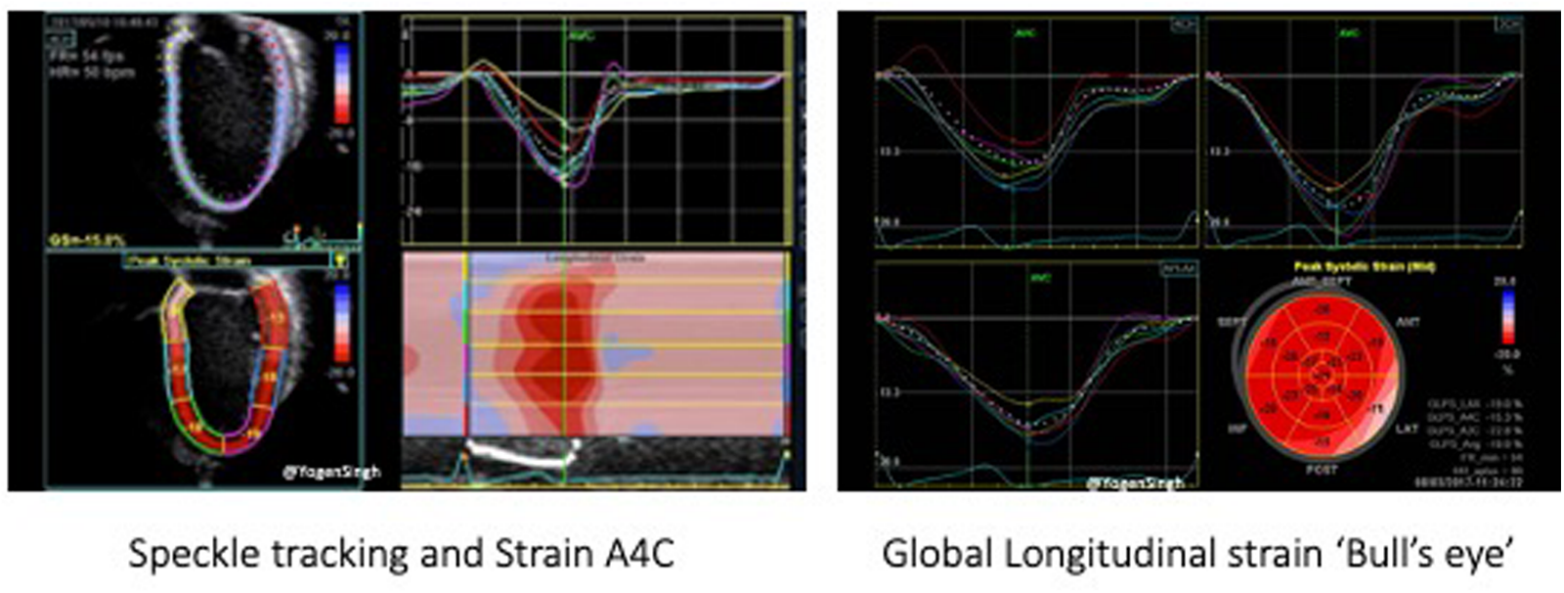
Myocardial strain measurements on two-dimensional speckle tracking echocardiography.
